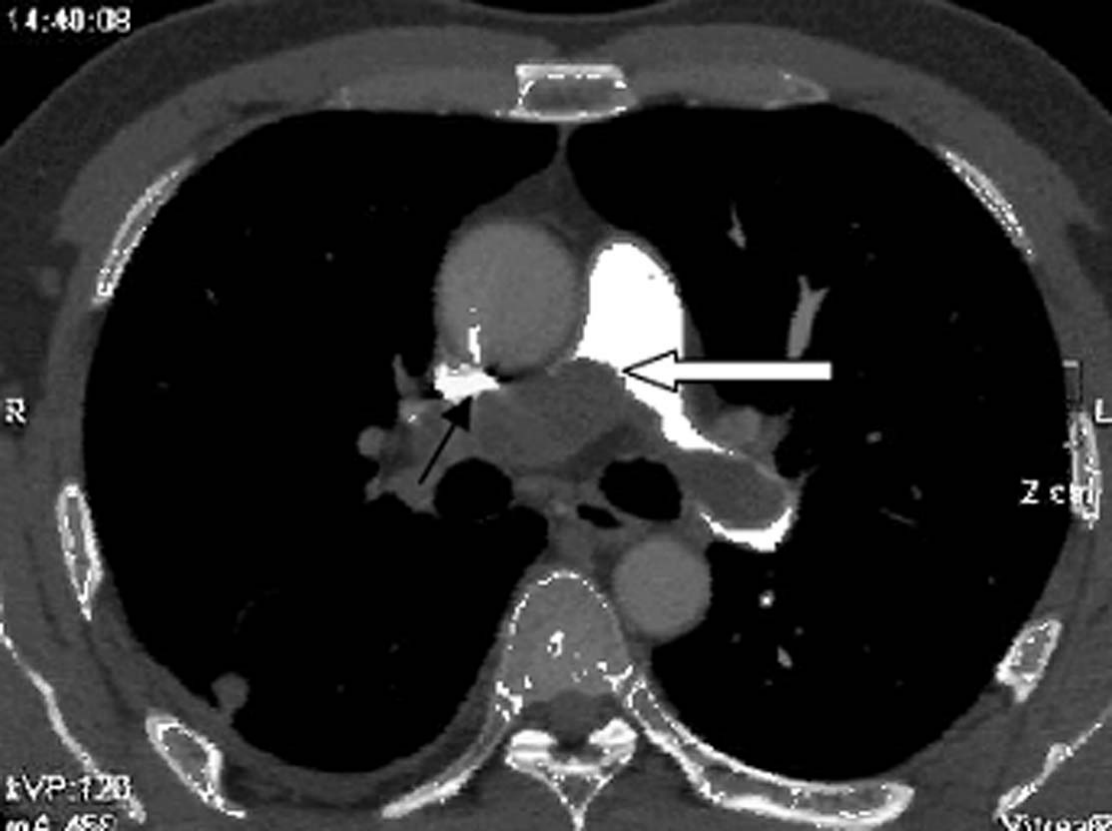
Pulmonary artery computed tomography angiography, axial view. The lumen of the pulmonary trunk is obliterated by a low-density mass (white arrow) that extends into the left and right pulmonary arteries. Both walls of the left pulmonary artery are eclipsed by the lesion (black arrow). The pulmonary trunk, left pulmonary artery, and right pulmonary artery are fully or partially occupied by the lesion, and the proximal end of the lesion protrudes towards the right ventricular outflow tract (white arrow). We termed this appearance the wall eclipsing sign.
